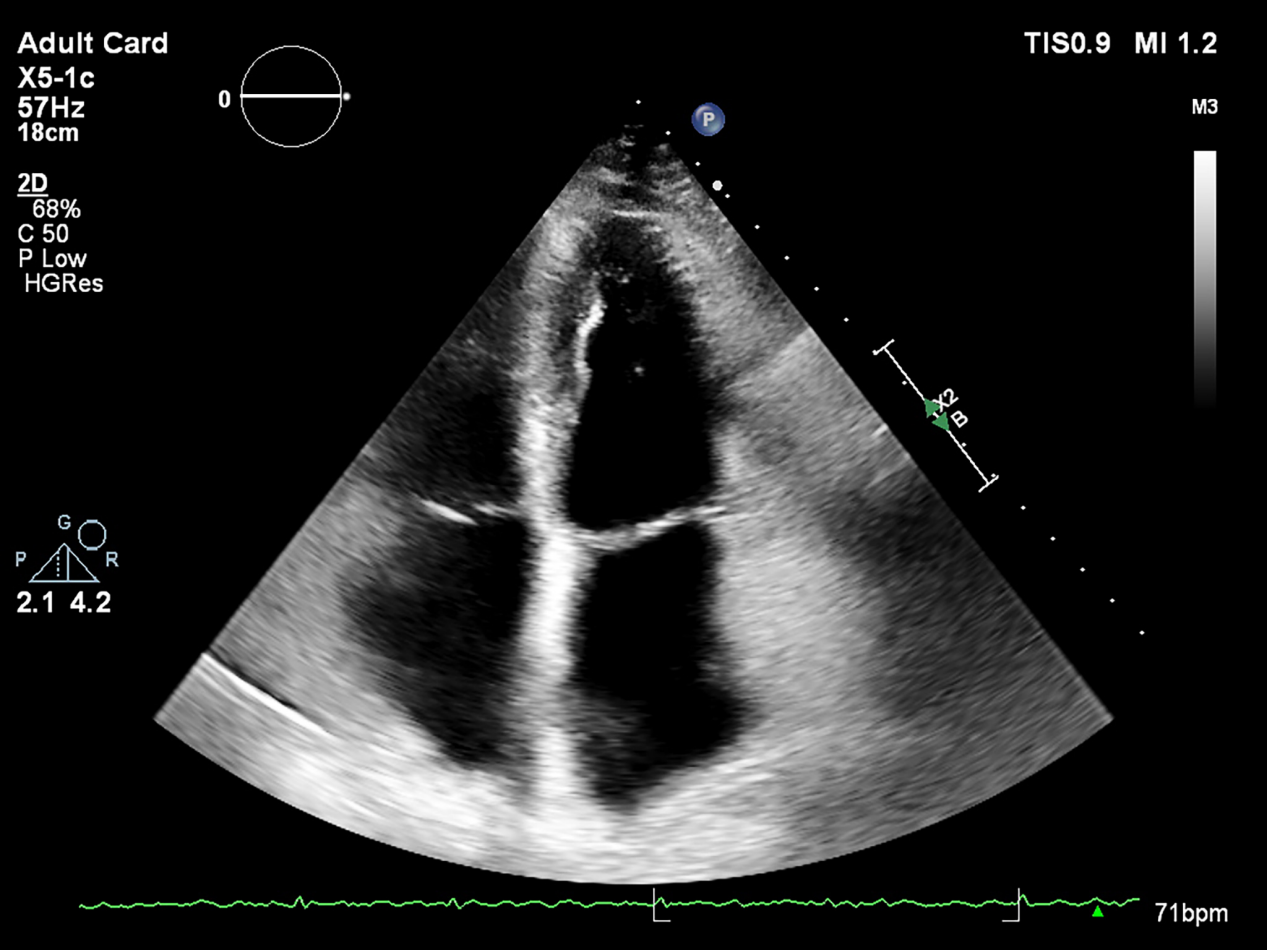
Bidimensional echocardiography – 4 chambers view, maximum left
